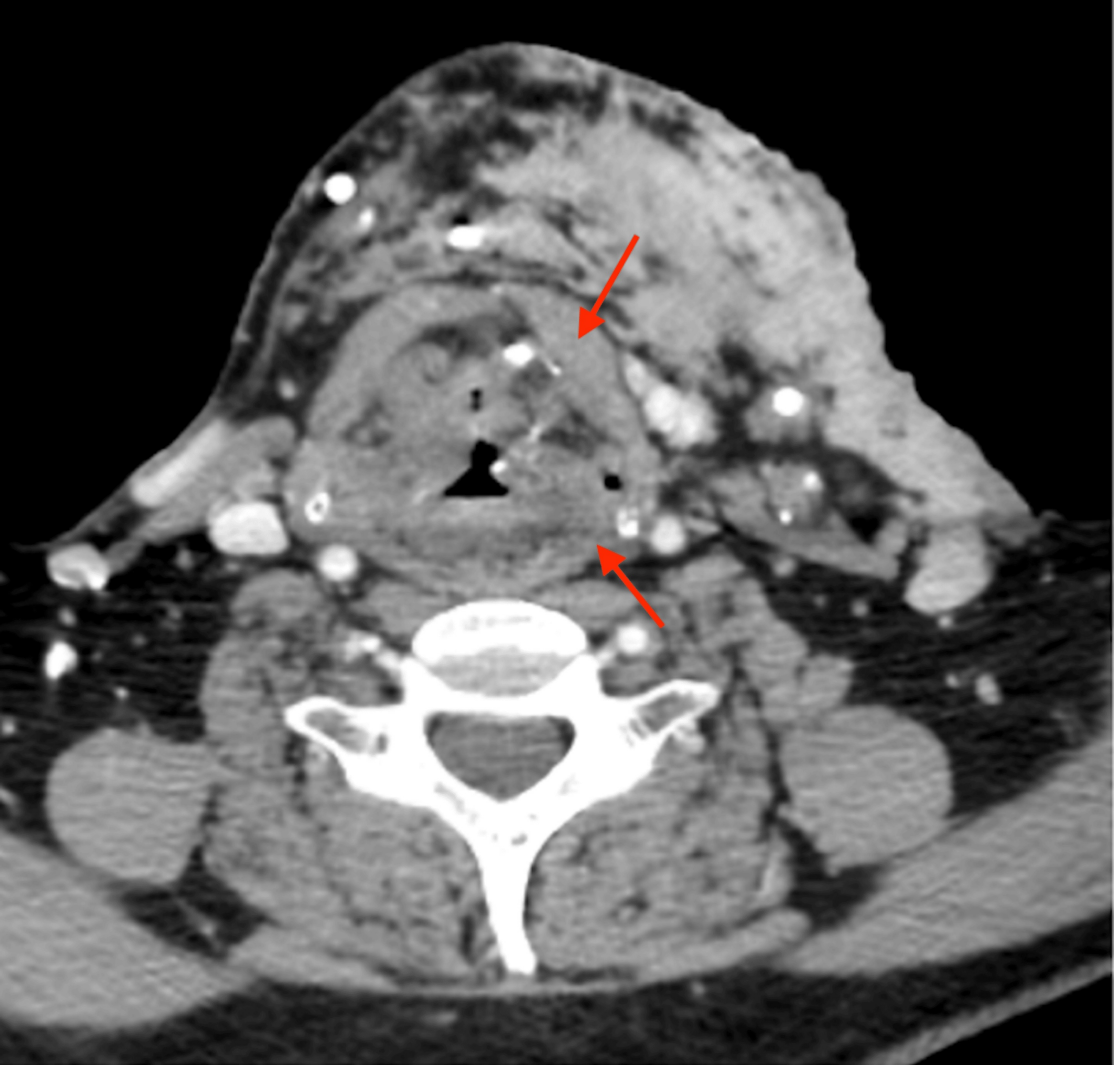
Axial view of a CT scan of the neck at the level of the larynx further delineating extension, as shown by the red arrows CT: Computed tomography
